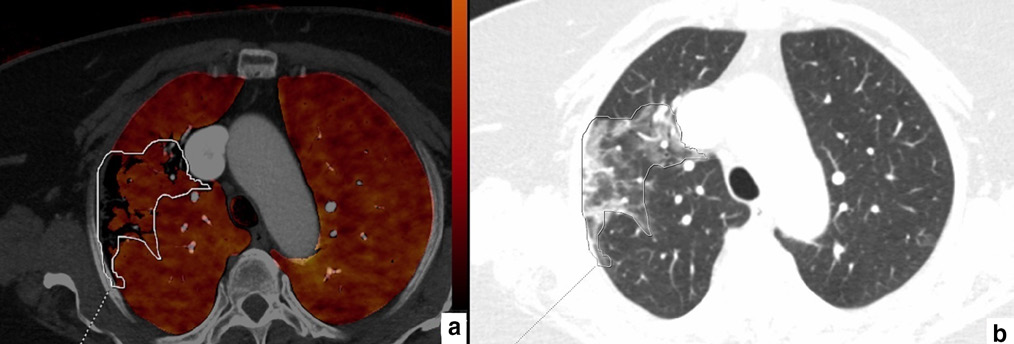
64-year-old female. Axial perfusion blood volume (a) and computed tomography (b) images. In the right upper lobe, a large, heterogeneous area of GGOs and consolidations can be seen (b, marked area). Perfusion deficits are also present in the same area, but they take up less space than GGOs and consolidations (a, marked area).
